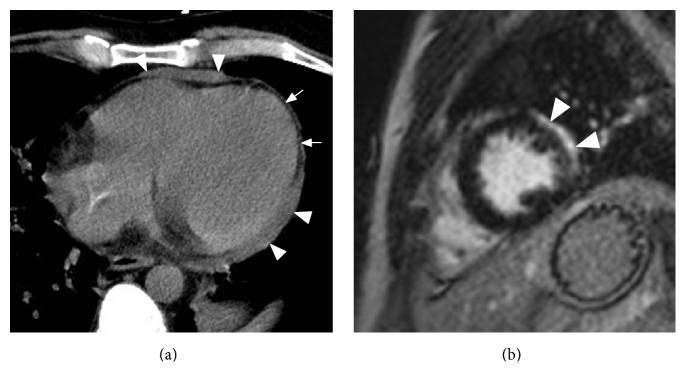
(a) Follow-up noncontrast CT after chemotherapy demonstrates marked treatment response with visualization of the pericardial fat* (arrows)* and mild residual soft tissue along the anterior RV and lateral LV* (arrowheads)*. (b) CMR DME performed for further characterization shows avid delayed enhancement of the soft tissue along the lateral LV* (arrowheads)*, compatible with posttreatment scar.
